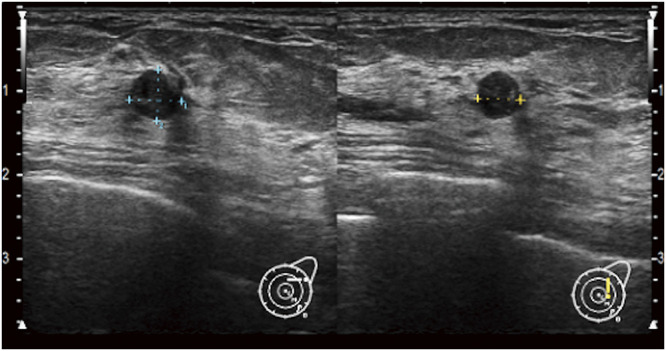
Ultrasound revealed a 6 mm, smooth, well-defined mass in the left C area.
